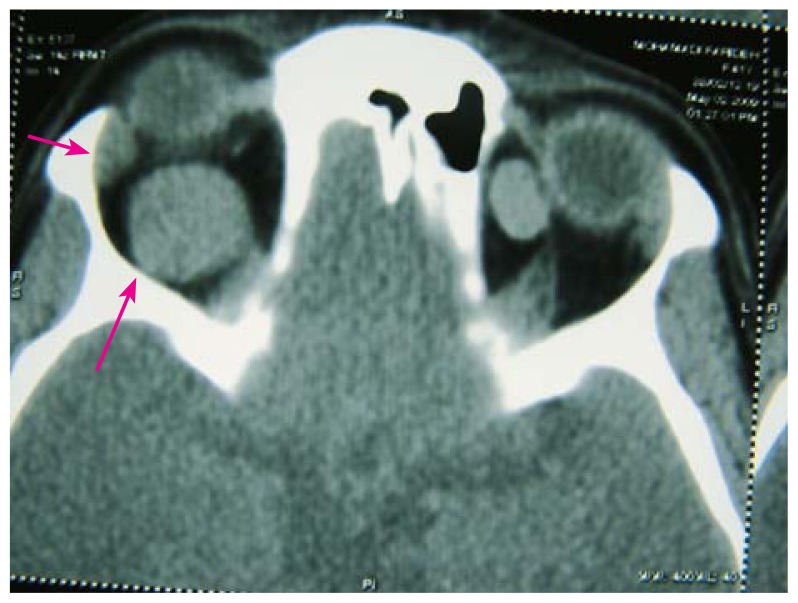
Orbital computed tomography revealed a well-defined round homogenous intraconal soft tissue mass with severe proptosis in the right orbit and a smaller lesion in the superomedial extraconal space of the left orbit.
